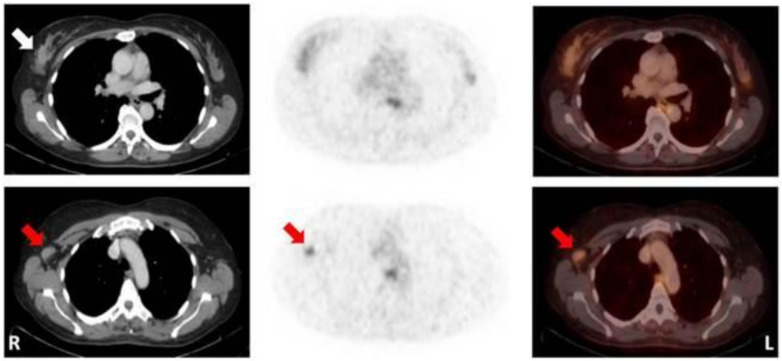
Patient with a mucinous carcinoma of the right breast with low ER/PR-expression. CT (left); ^68^Ga-RM2-PET (middle); fusion images (right); primary tumour indicated by white arrow; lymph node metastasis indicated by red arrows. (Figure unmodified from Stykow et al. [79] Creative Commons Attribution 4.0 International License).
